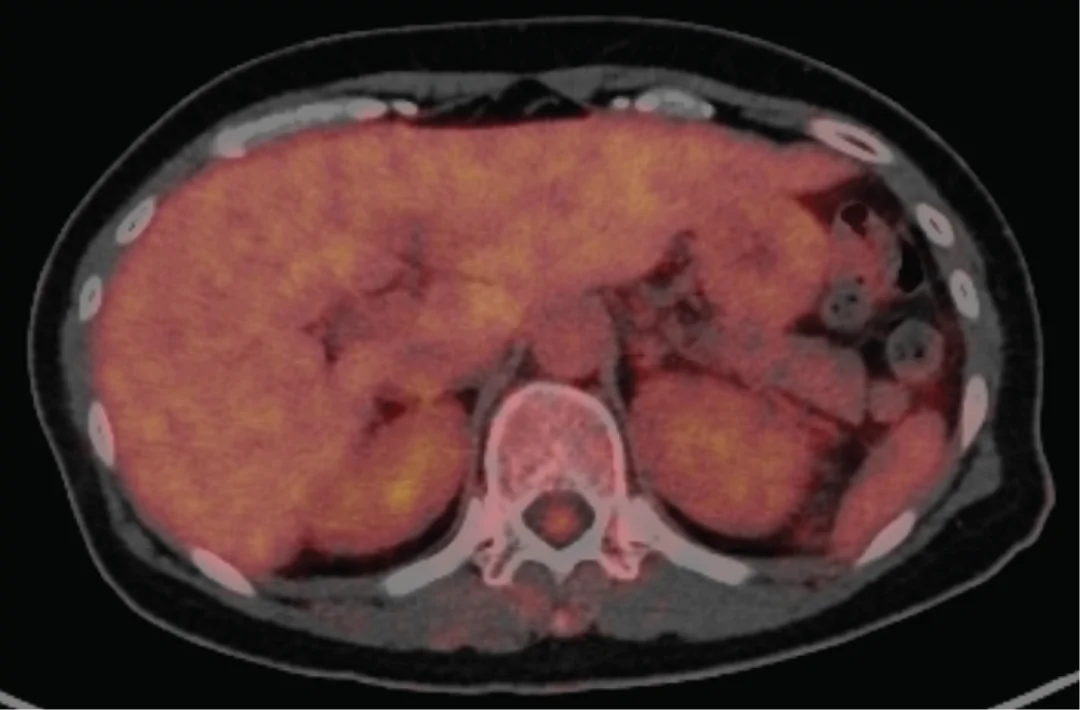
FDG-PET/CT findings after completion of T-DM1 therapy. No abnormal FDG uptake was observed in the hepatic lesions corresponding to the nodules detected on EOB-MRI, supporting the absence of metabolically active metastatic disease. EOB-MRI, gadolinium-ethoxybenzyl-diethylenetriamine pentaacetic acid-enhanced MRI; FDG, fluorodeoxyglucose; T-DM1, trastuzumab emtansine
